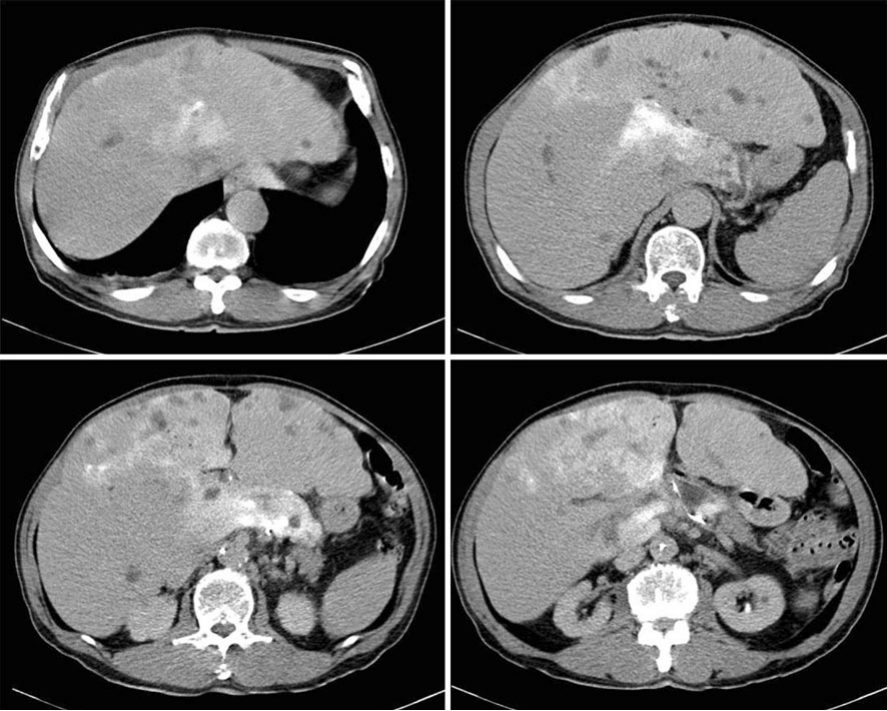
Non-contrast CT immediately after TAE Bright area showed pooled gelatin particles. The area of pooled gelatin particles was very limited compared to the total volume of primary tumor in left and middle hepatic lobe
